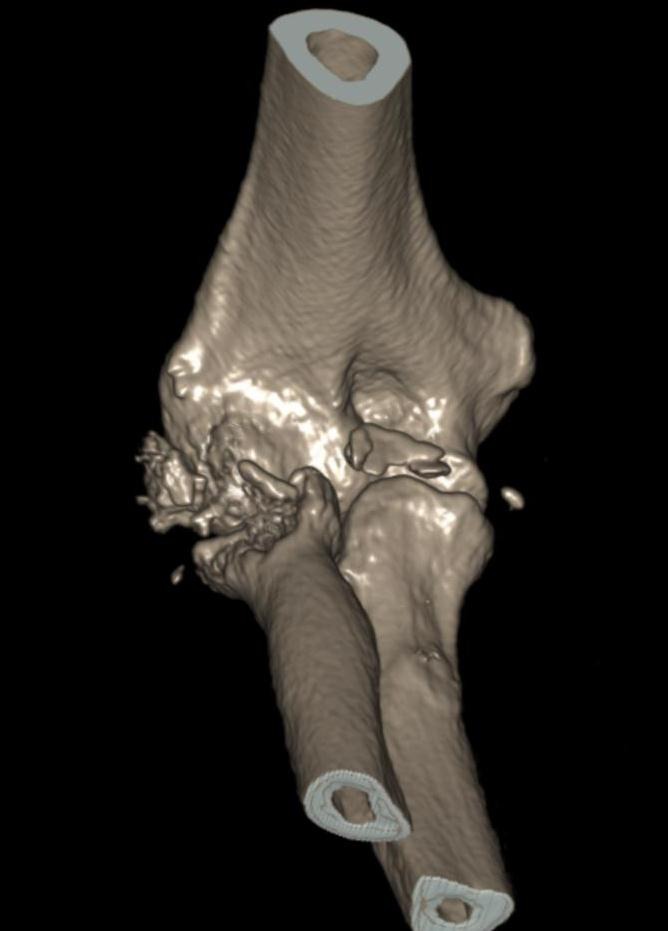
The CT scan shows a comminuted radial head fracture and coronoid fracture.
